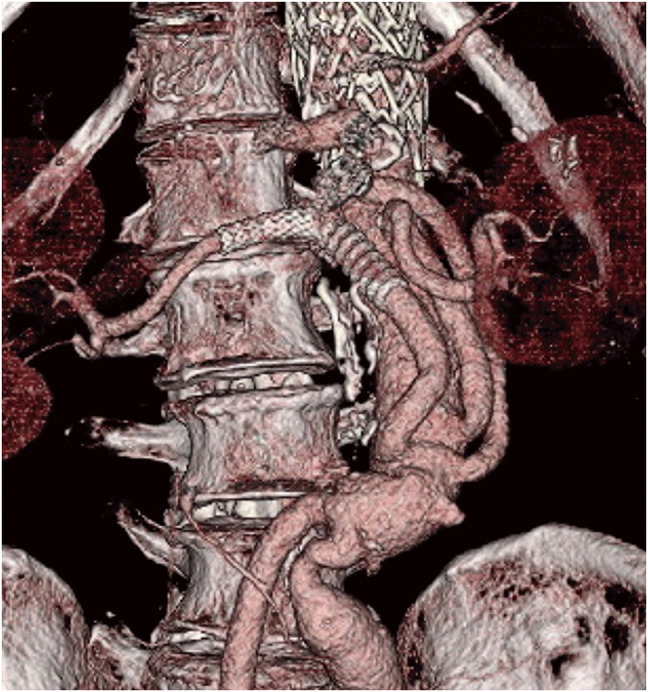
Three-dimensional cardiac computed tomography angiography showing the disappearance of the aneurysm after stent-graft implantation.
